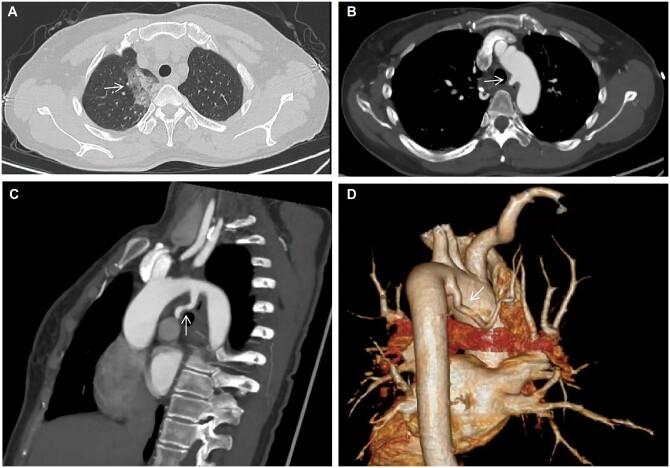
(A) Chest computed tomography. (B, C) Computed tomography angiography in axial and sagittal views. (D) Reconstructed three-dimensional computed tomography angiography. Arrow demonstrates (i) flake ground-glass shadows in the right upper lobe. (ii) An aberrant artery arises from the aortic arch.
